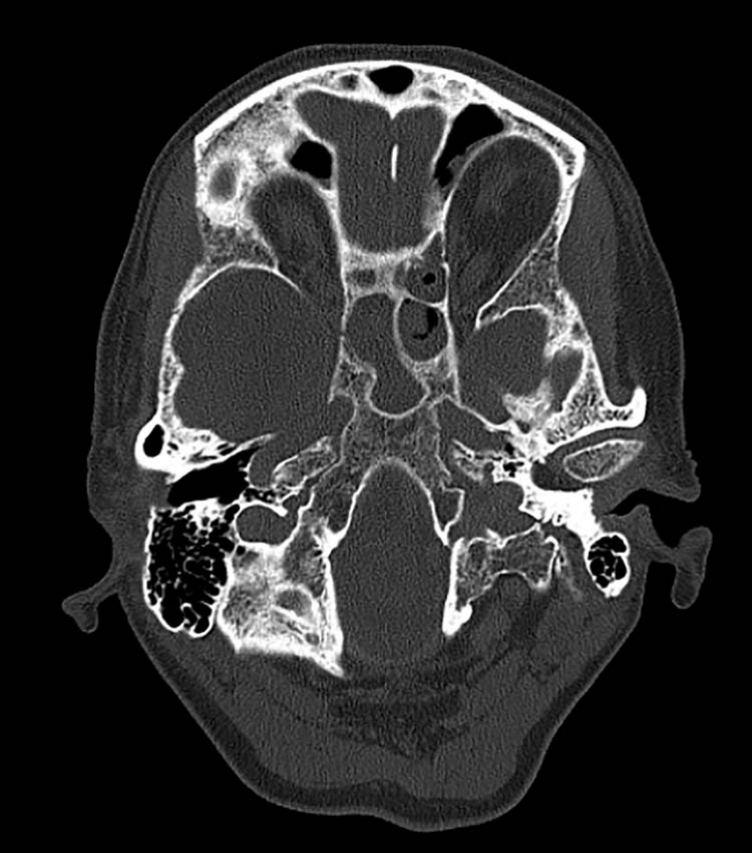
CT head revealed no signs of meningeal or intracerebral enhancement to suggest an intracranial abscess or cerebritis, but it suggested significant opacification in the paranasal sinuses, particularly in the ethmoid and sphenoid sinuses, most likely indicating a minor post-operative haemorrhage.
